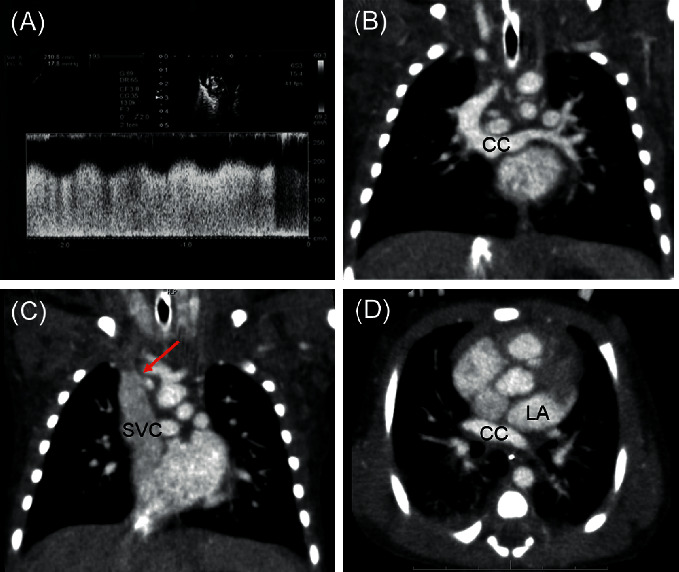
Various tests after birth. (A) Echocardiography displays a continuous flow pattern at the confluence of the pulmonary vein common chamber with the superior vena cava. (B) Contrast-enhanced computed tomography reveals bilateral pulmonary veins flowing into the common chamber. (C) The red arrow indicates severe stenosis of the confluence of the pulmonary vein common chamber with the superior vena cava. (D) The common chamber did not connect with the left atrium.
